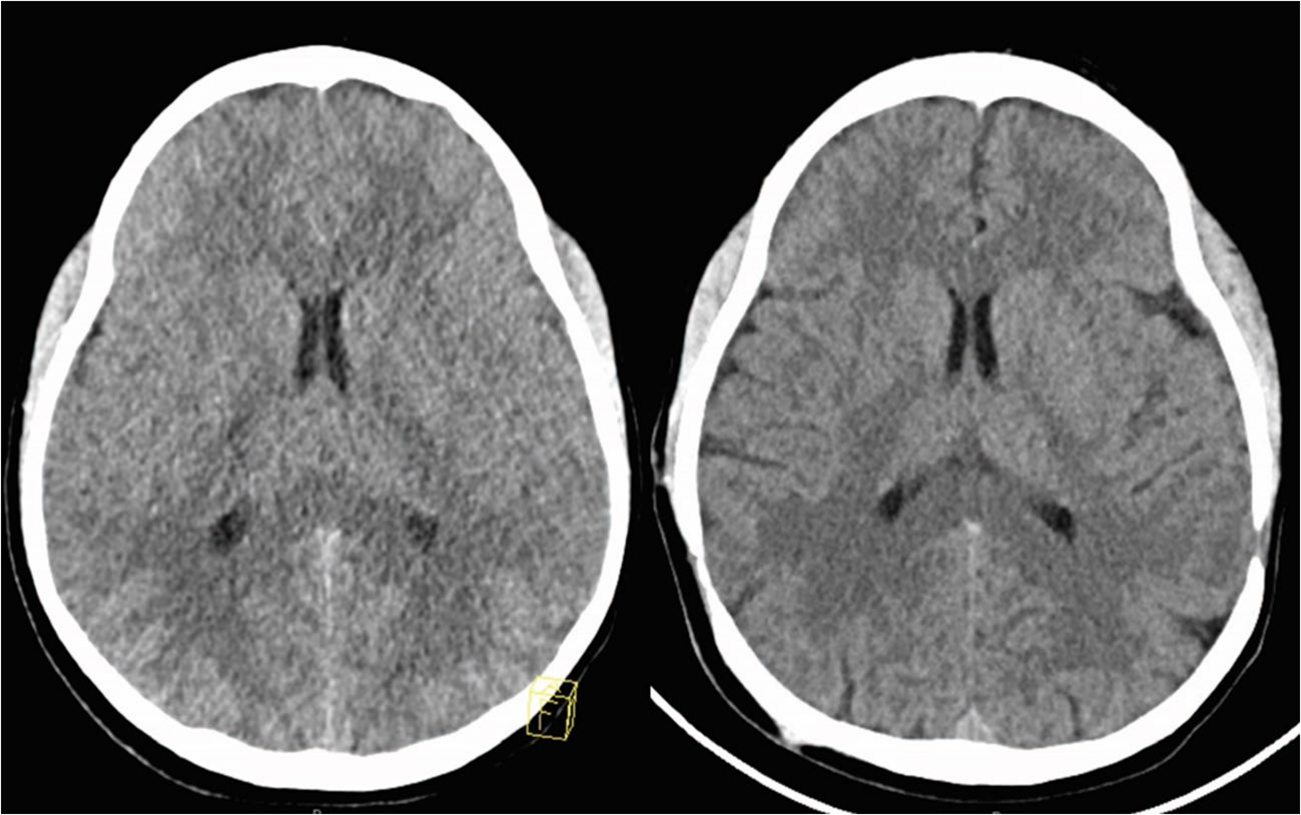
Computed tomography (CT) scan of a patient with idiopathic intracranial hypertension (IIH) a prior to and b following posterior vault distraction osteogenesis (PVDO)
